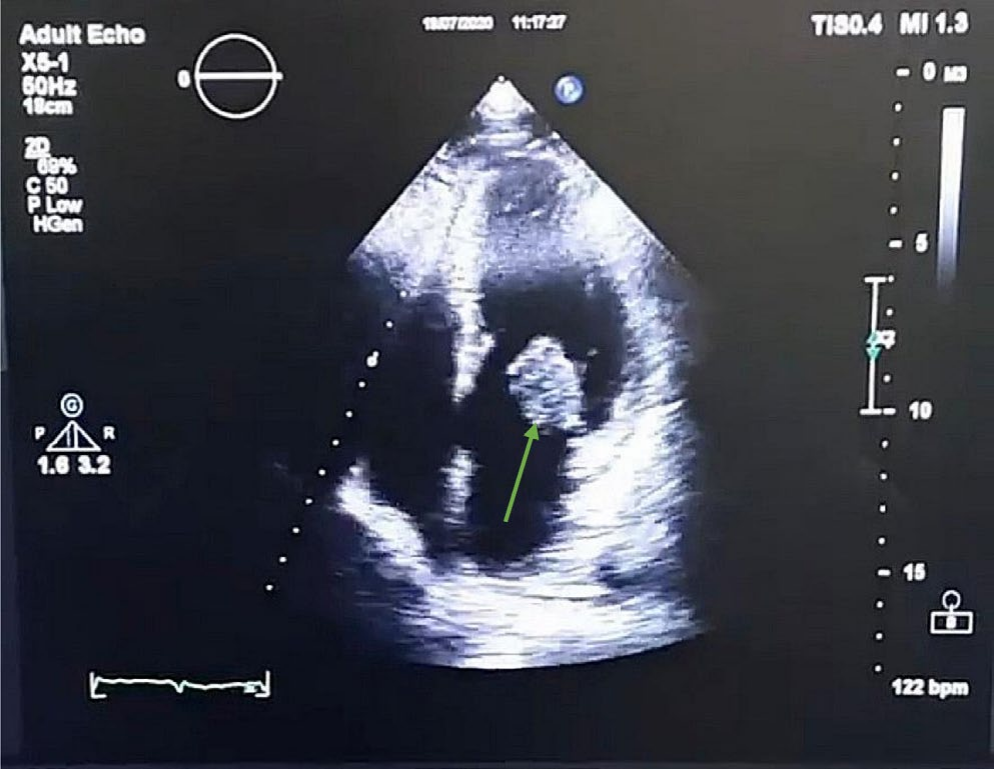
Echocardiographic image before the patient's heart surgery. A vegetation of about 2.5 cm was observed on the posterior leaflet of the mitral valve, which is marked by green arrow.
